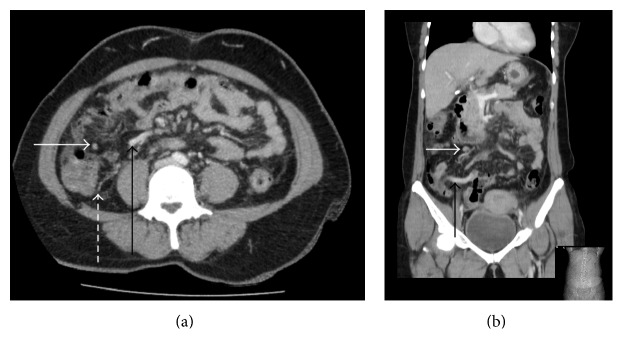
(a) Axial CT with intravenous contrast. The appendix is seen anterior to the right psoas muscle belly and is with a little surrounding free fluid (solid black arrow). Increased attenuating soft tissue in the right paracolic gutter suggests free fluid or secondary peritoneal metastases (broken arrow). (b) Coronal section of the same patient demonstrating the enhancing appendix medial to the caecum. Mural enhancement is commonly seen in appendicitis. However, in this case, there is little surrounding fat stranding and no fluid filled lumen is seen; these features are more atypical. Enlarged lymph nodes are seen in the mesentery (solid white arrow).
